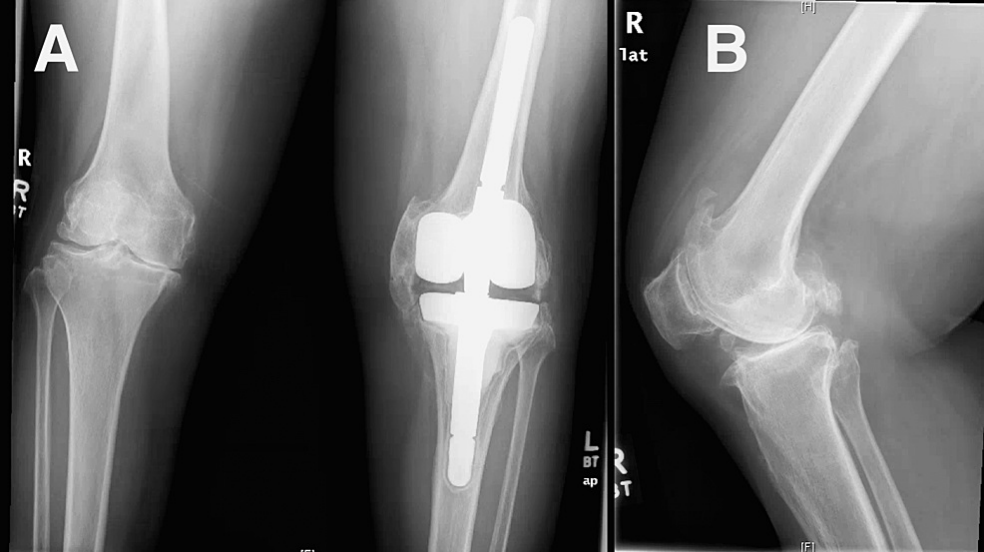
Preoperative bilateral anteroposterior (A) and right lateral (B) radiographs demonstrating osteoarthritis.
